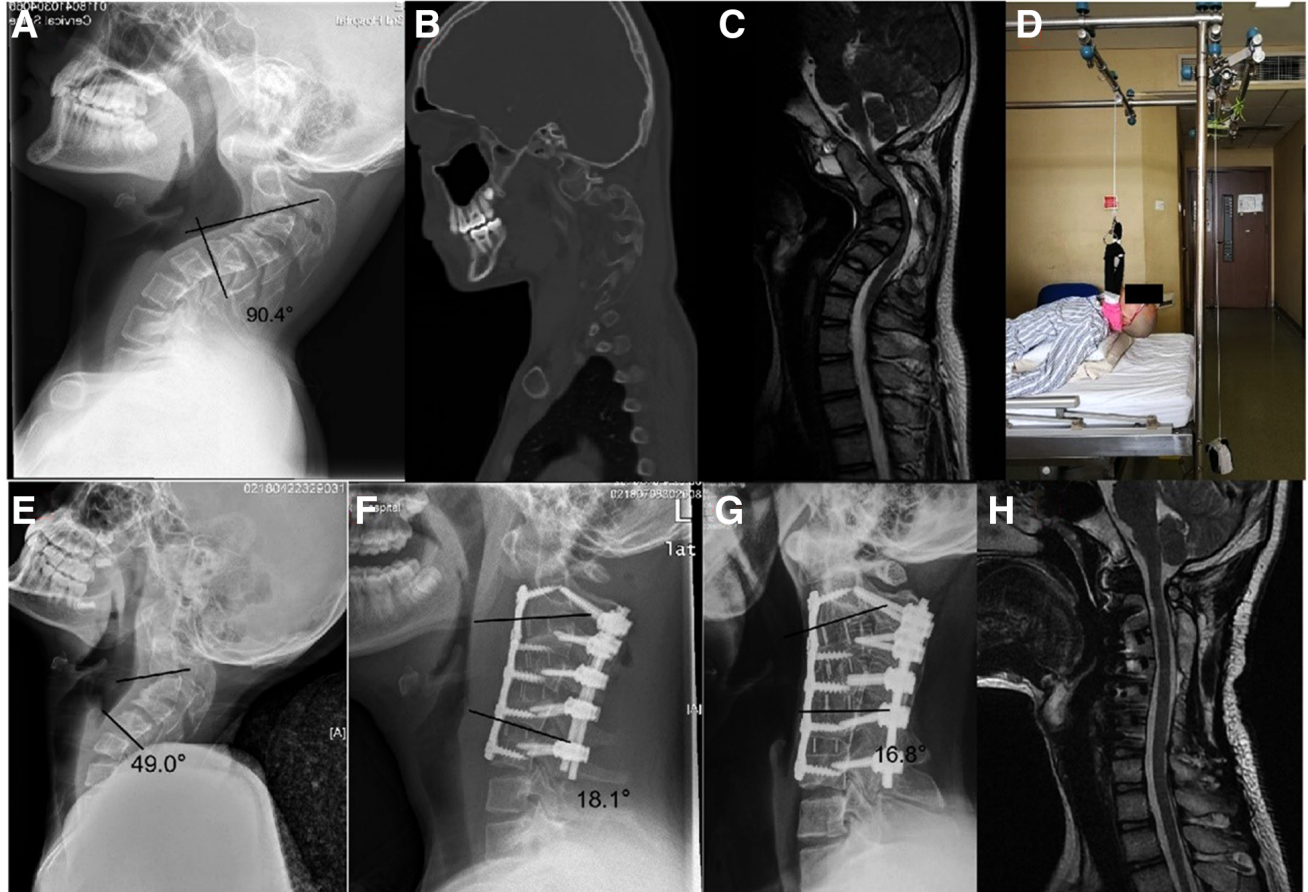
Case 2. This 14-year-old male complained of neck pain and weakness of the hands due to myelopathy caused by idiopathic cervical kyphotic deformity. (A) Pre-operative x-ray revealed a C2–5 Cobb angle of 90.4° before traction. (B) A para-sagittal computed tomography (CT) reconstruction showed C3–4 facet joint fusion. (C) Sagittal magnetic resonance imaging (MRI) showed severe compression of spinal cord at C2–4 level. (D) One week suspensory traction. (E) After one week of suspensory traction, the Cobb angle had reduced to 49°; the correction rate was 45.8%. (F) Post-operative x-ray showed the Cobb angle reduced to 18.1°. (G) x-ray at 1 year showed no loss of correction. (H) MRI showed completed decompression of cervical spinal cord with the modified mJOA score improved from 14 to 17 post-operatively.
